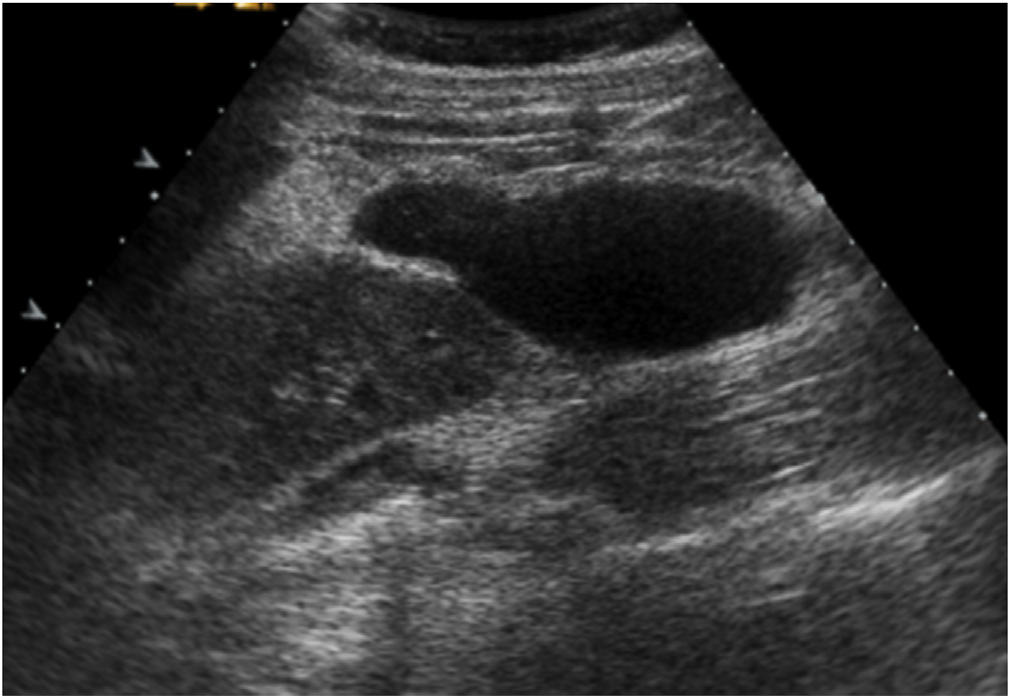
Pre-operative ultrasound.
